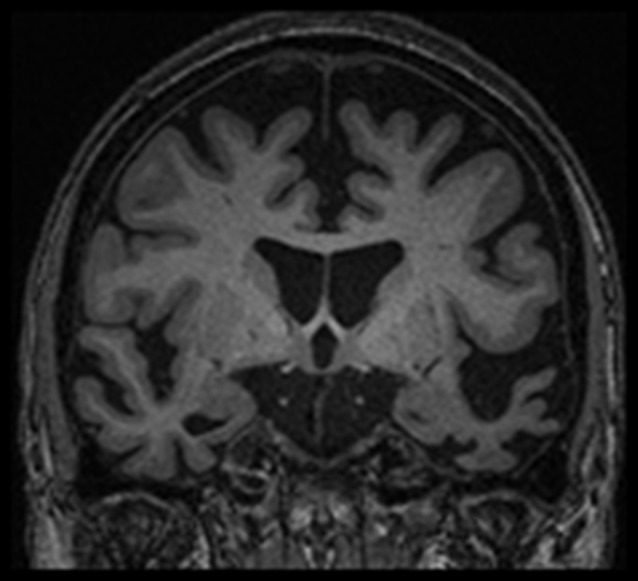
Magnetic resonance imaging for the patient from fall 2013, showing predominantly left-hemispheric cortical atrophy, most prominent in the anterior temporal lobe. Shown as per radiological convention (left side on right).
